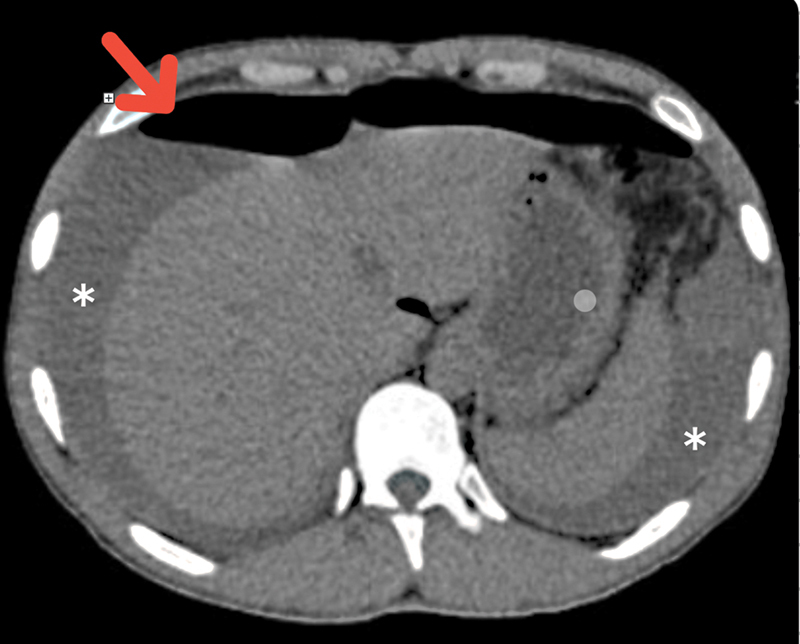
Abdominal computed tomography (CT) scan. Free intraperitoneal air, free fluid surrounding the spleen and liver (the arrow signifies the free intraperitoneal air).
